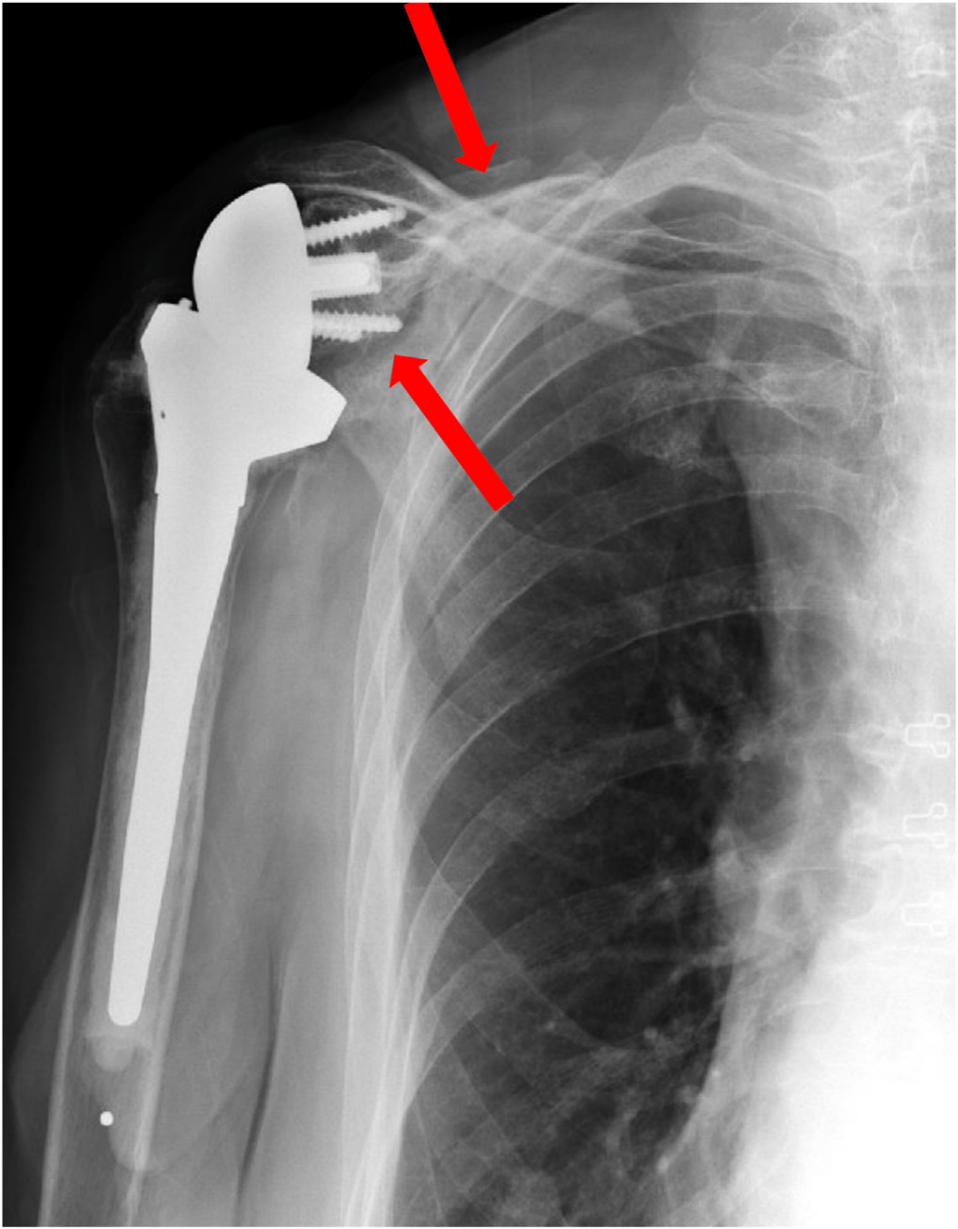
X-ray Grashey view demonstrating healing of the scapular body fracture.
